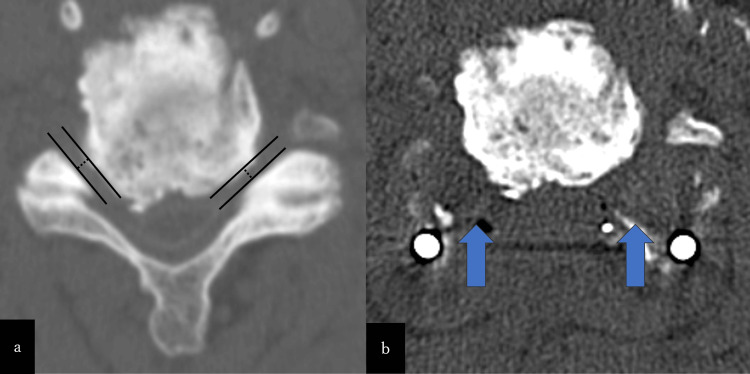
Axial CT at C4/5 (a) Preoperative image showing bilateral foraminal diameters measured at the narrowest point. (b) Post-facetectomy image showing a widened foramen on the operated side after facet resection.
